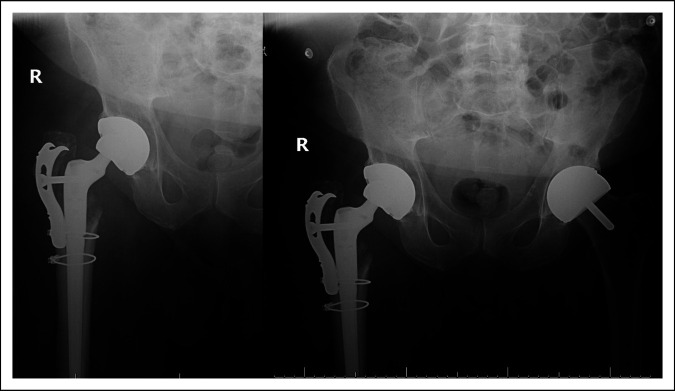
Preoperative second revision radiographs on May 09, 2021.
